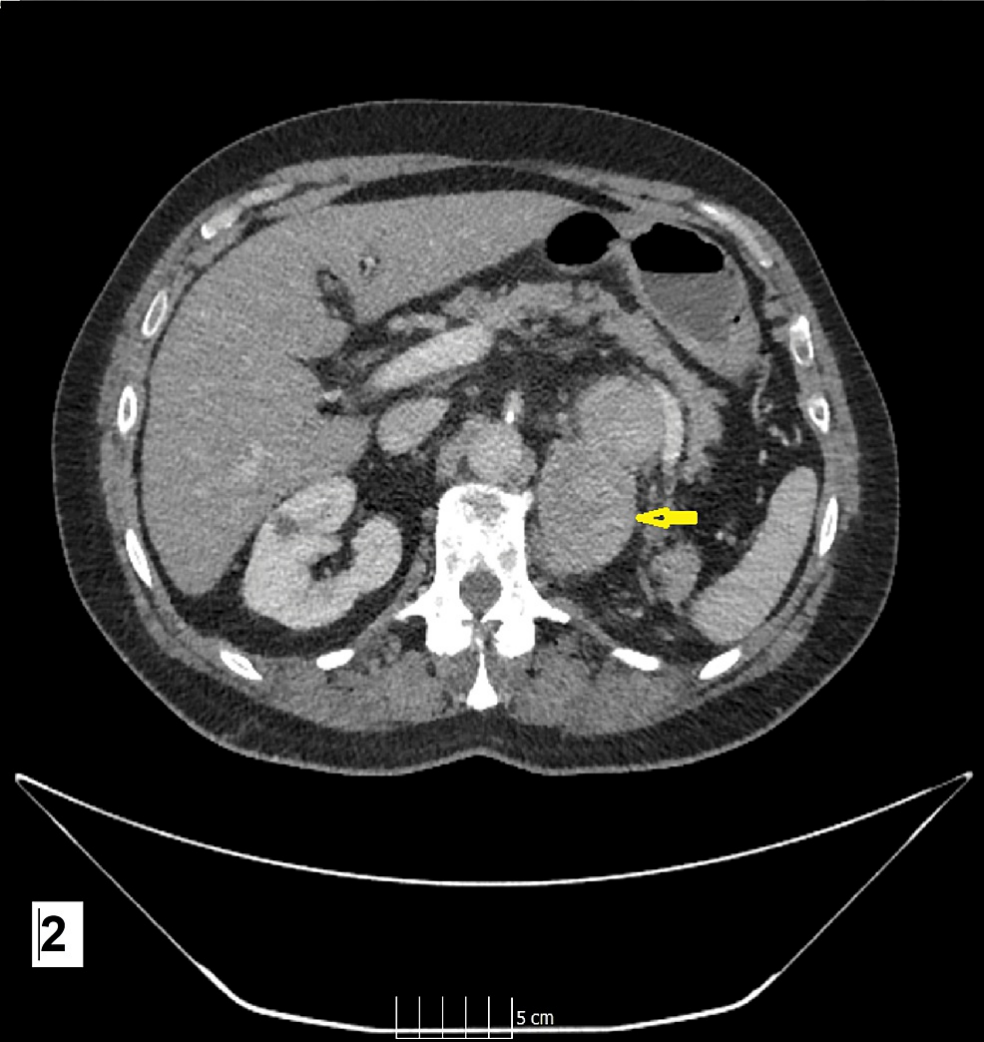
Axial image of the CT scan showing the tumour recurrence site The yellow arrow points to the left nephrectomy bed recurrence.
